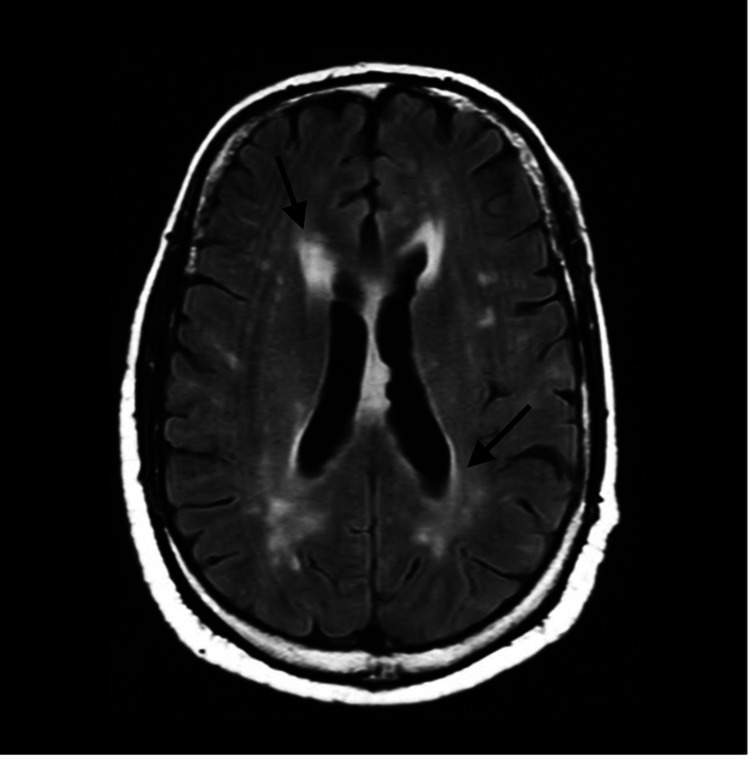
MRI of the brain on admission MRI: Magnetic resonance imaging Fluid-attenuated inversion recovery (FLAIR) sequences demonstrating confluent areas and foci of high signal in periventricular and deep white matter (black arrows).
